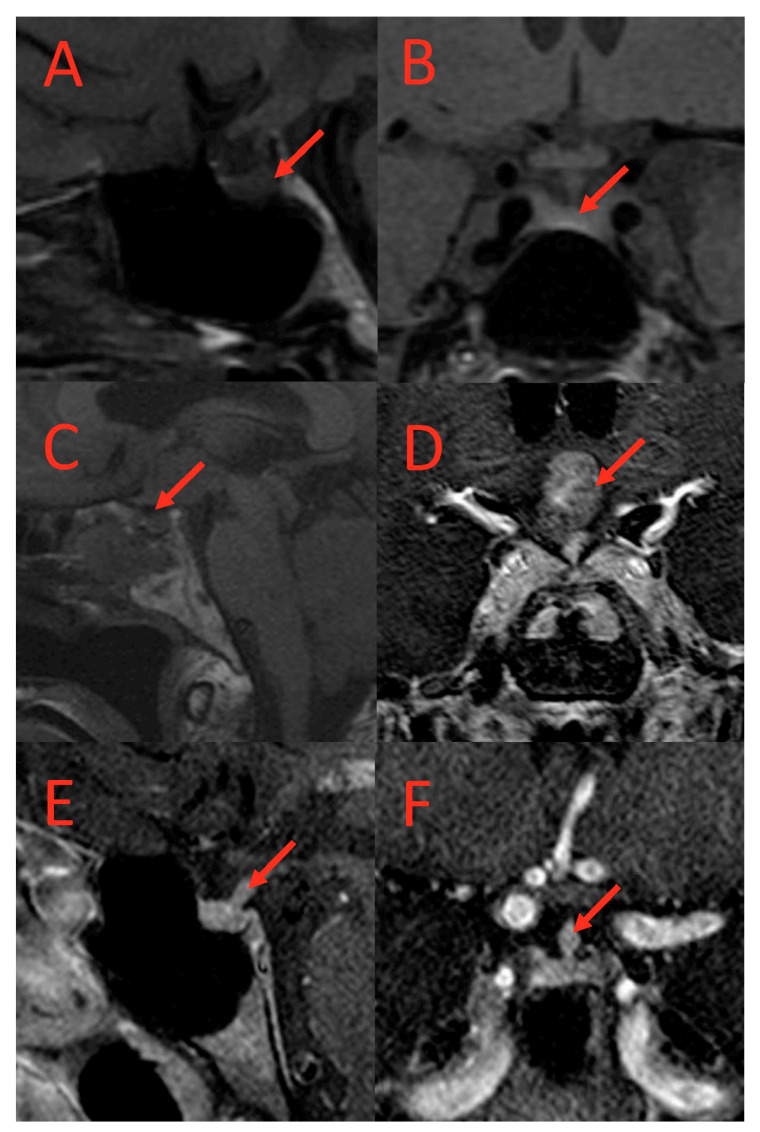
T1-weighted MRI scans of subjects with AVP-D. (A) (coronal) and (B) (sagittal): Loss of posterior pituitary bright spot in a subject with AVP-D. (C,D): Suprasellar mass in a subject with DI. (E,F): Thickened pituitary stalk at hypothalamus in a subject with AVP-D and loss of posterior pituitary bright spot. Red arrows point to the lesion.
